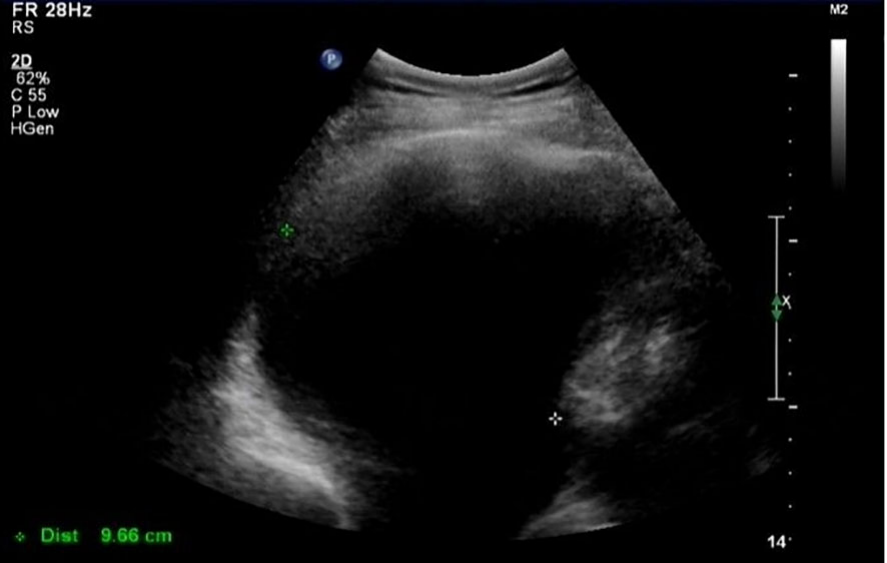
Ultrasound image of pleural effusion.
